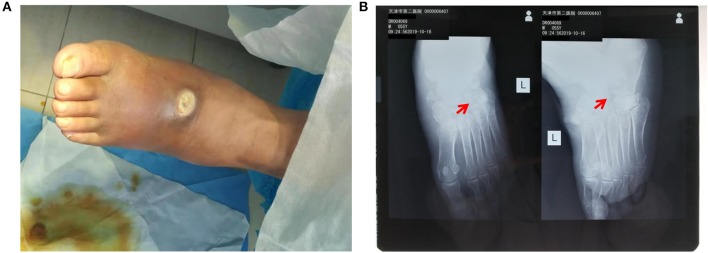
Pre-operative detection showed swelling and intermediate cuneiform displacement in the left foot. (A) Distinct swelling and 2 × 3-cm sized ulcer presented on the surface of left-foot dorsum; (B) X-ray detection revealed intermediate cuneiform fracture and displacement. The red arrows indicate the position of displaced intermediate cuneiform.
